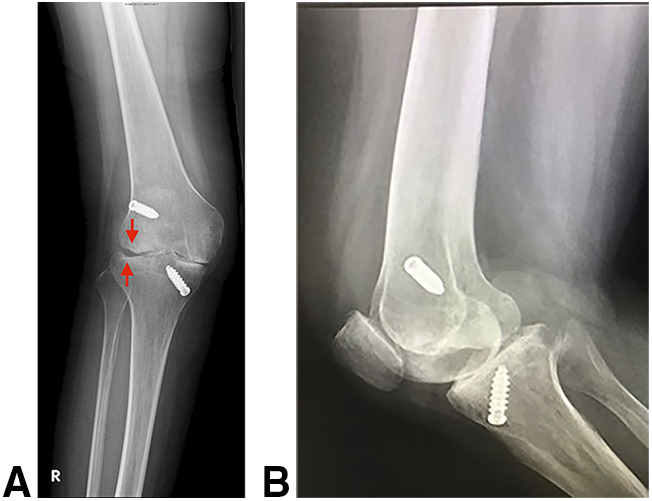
Preoperative radiograph of the right knee showed bone loss over the lateral femoral condyle and lateral tibial plateau depression fracture (A) and posterior migration of the tibia (B)
